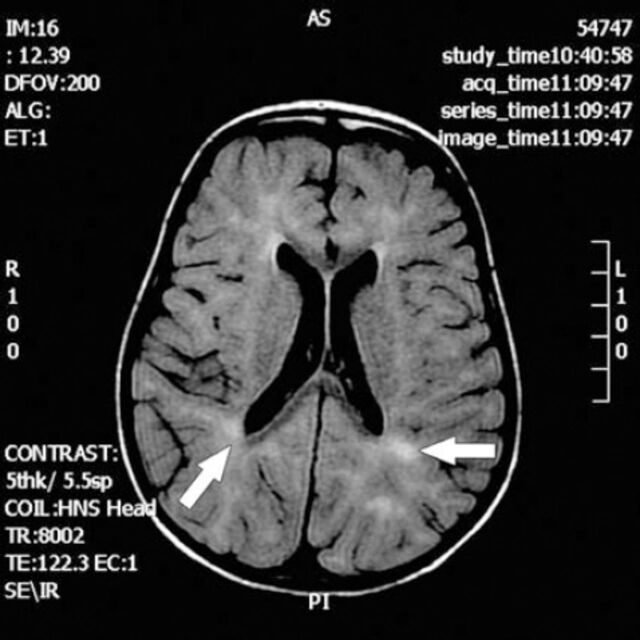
Magnetic resonance imaging (Flair) of a 5 years and 8 month-old male patient with cerebral folate deficiency showing normal myelin signal of callosal structures with decreased myelin signals for white matter and loss of myelin signals around occipital horns.
